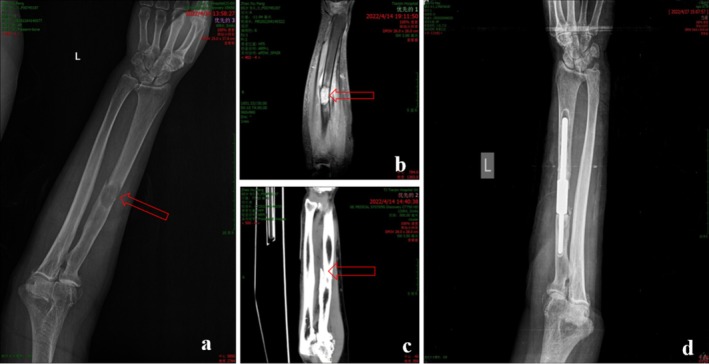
The diaphyseal mortise‐tenon prosthetic reconstruction for pathological fracture of the left radial diaphysis in patient 1. (a and b) Preoperative X‐ray and magnetic resonance images show metastatic lesion in the left radius (*arrows*). (c) Preoperative computed tomography scan shows osteolytic destruction, discontinuity of the bony cortex, an obvious fracture line, and angulation deformity in the left radial diaphysis (*arrow*). (d) Postoperative X‐ray image shows that the prosthesis is in good position. All images have been anonymized to protect patient privacy.
